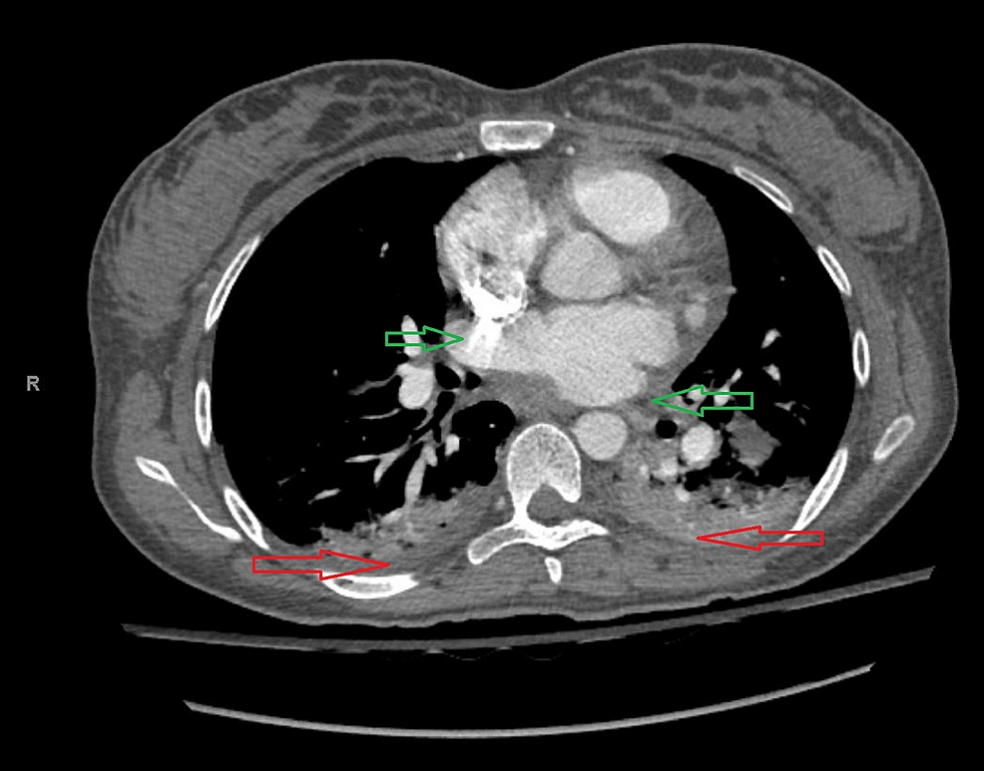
CT pulmonary angiogram showing bilateral pleural effusions with no evidence of pulmonary embolism. CT: computerized tomography; red arrows: pleural effusions; green arrows: pulmonary arteries
